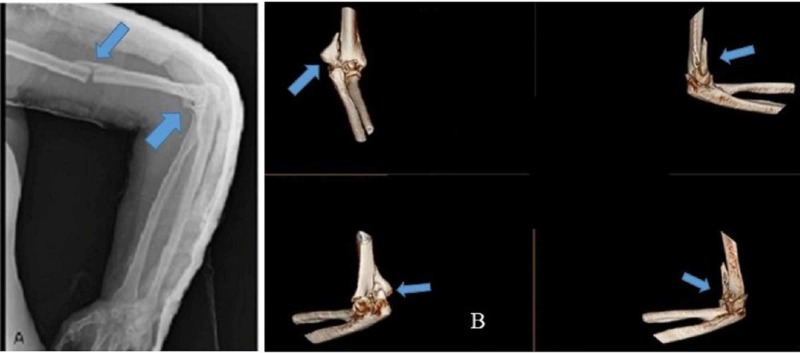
Preoperative radiograph showing the left shaft of humerus fracture with a concomitant distal humerus fracture (A). Computed tomography scan of the left distal humerus showing OTA/AO-13-C2 (Orthopaedic Trauma Association/Arbeitsgemeinschaft für Osteosynthesefragen) fracture with a Type III capitellar fracture (B).
